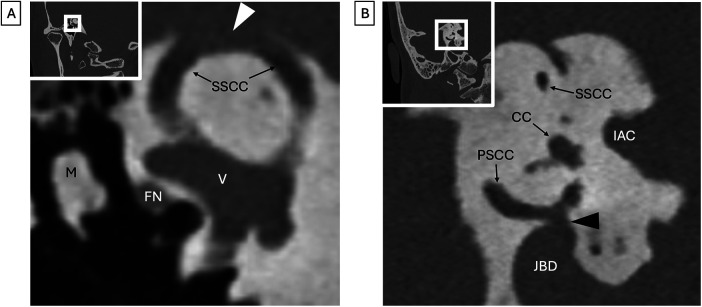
Third window defects (both images represent different patients). (A) Photon-counting Pöschl view CT shows dehiscence (arrowhead) along the roof of the superior semicircular canal (SSCC). Vestibule (V), head of the malleus (M), and tympanic facial nerve canal (FN) are provided for reference. (B) Photon-counting Stenvers view CT shows dehiscence (arrowhead) of the posterior semicircular canal (PSCC) adjacent to a jugular bulb diverticulum (JBD). Superior semicircular canal (SSCC), common crus (CC), and internal auditory canal provided for reference.
